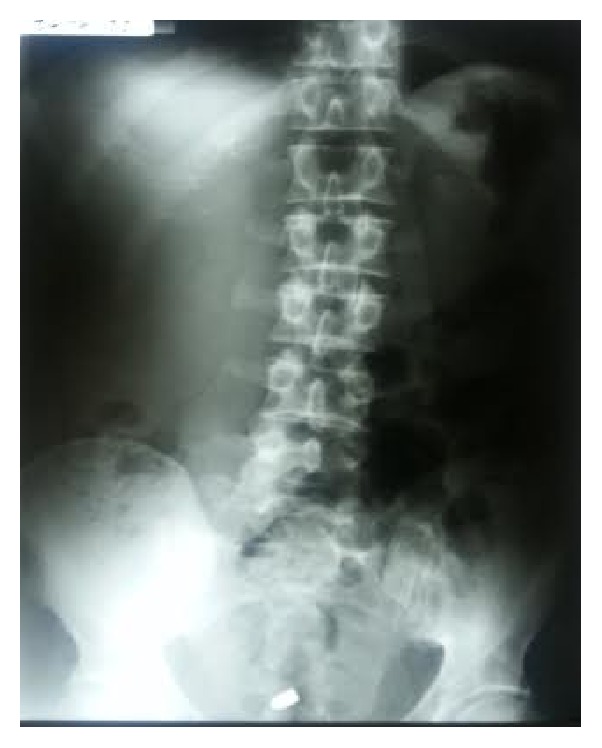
Erect abdominal radiograph shows the bullet in the lower abdomen.
